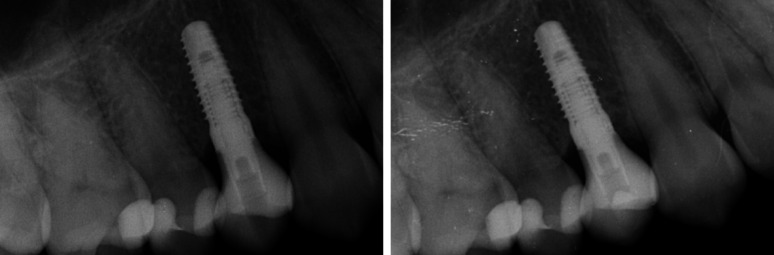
Radiographs of a PICN-ISC at prosthetic delivery (left) and follow-up (right)
